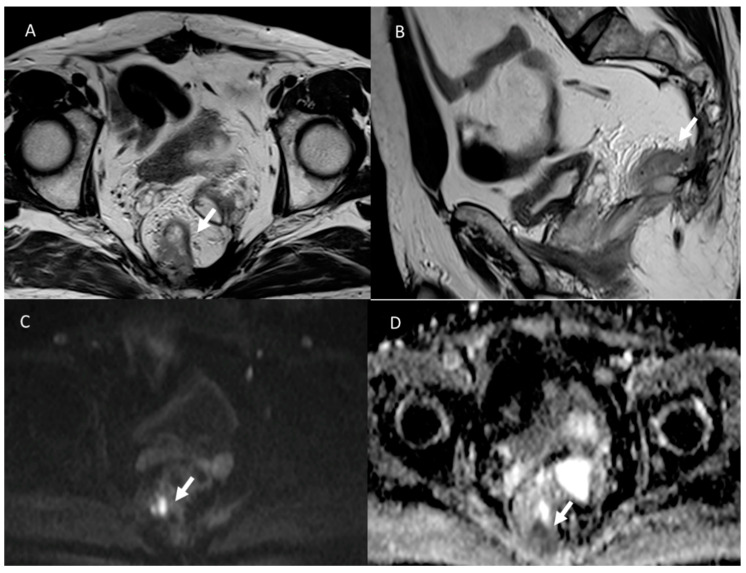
T2-W in sagittal plane (A) and T1-W post-contrast in sagittal plane (B) sequences of MRI assessment of post-surgical anastomotic leak. Arrows show a loss of continuity of the intestinal wall at the anastomosis site, with communication between the intra- and extra-luminal compartments. In DWI sequences (C: b800s/mm^2^ and D: ADC map), the lesion shows restricted diffusion.
